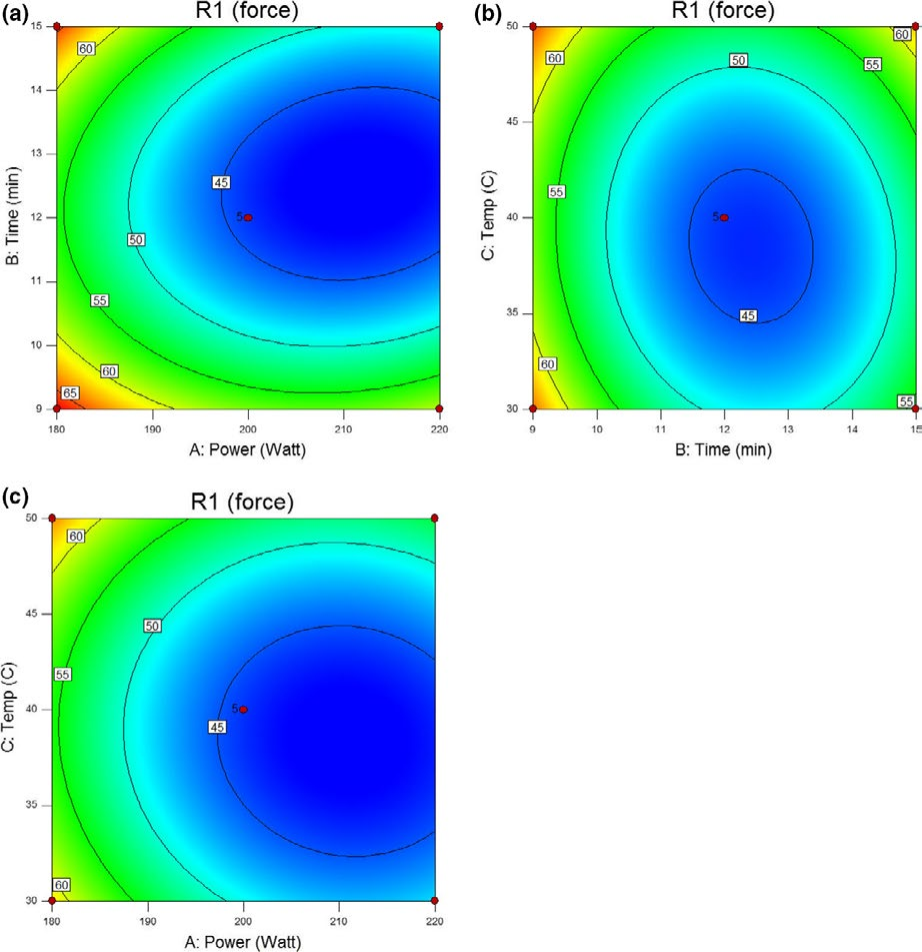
Two‐dimensional (2‐D) contour plots for the shear force of whelk meat subjected to the ultrasonic treatment with three variables (X1: ultrasound power, X2: treatment time, and X3: temperature). Contour plots showing the effect of (a): ultrasound power and treatment time; (b): ultrasound power and temperature; (c): treatment time and temperature on the shear force of whelk meat
